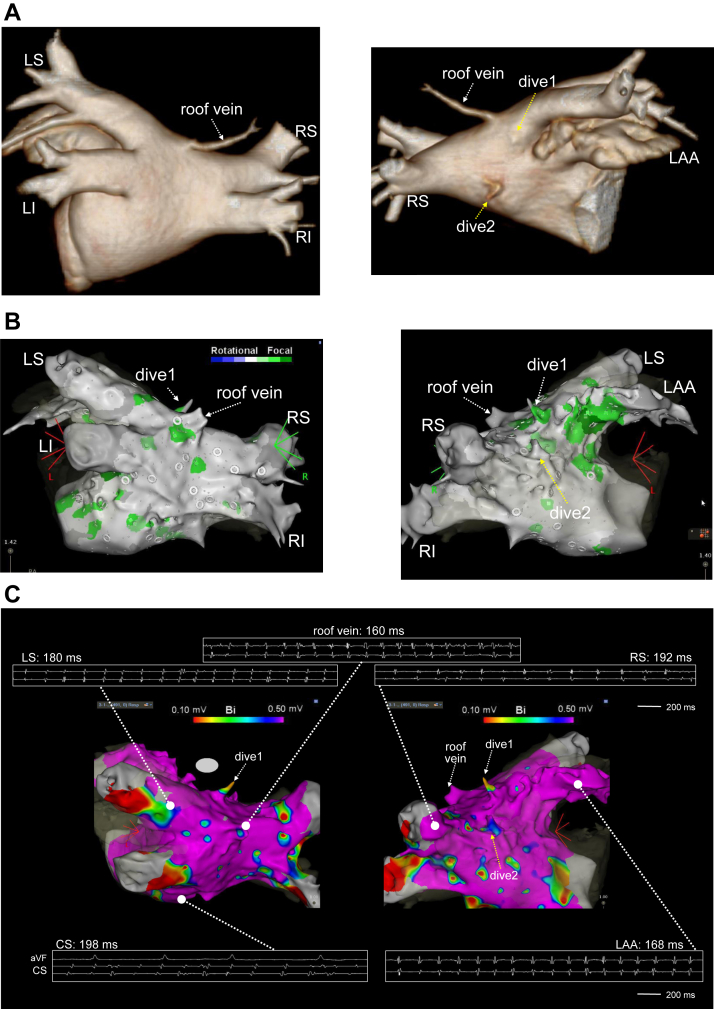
A: Three-dimensional computed tomography images showed a left atrial (LA) roof vein at the middle of the LA roof. In addition, there were 2 diverticula in the LA (dive1 and dive2). B: CARTOFINDER (Biosense Webster, Inc, Diamond Bar, CA) module map before pulmonary vein (PV) isolation. Drivers with a focal repetitive activation pattern (labeled in green) were identified in the LA appendage, PVs, septum, dive1 and the LA posterior wall, and around the roof vein. C: Voltage map in the LA during atrial fibrillation and cycle length at each site. The bipolar peak-to-peak voltages were >0.5 mV at the tops of dive1 and dive2. CS = coronary sinus; dive = diverticulum; LAA = left atrial appendage; LI = left inferior pulmonary vein; LS = left superior pulmonary vein; RI = right inferior pulmonary vein; RS = right superior pulmonary vein.
